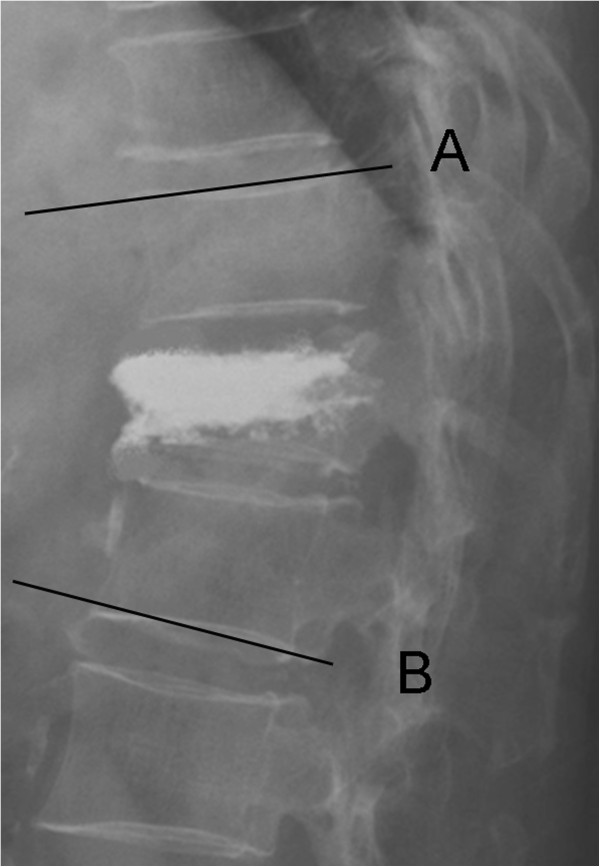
The balloon is deflated and withdrawn, and the resulting intravertebral cavity is filled with PMMA cement. Kyphotic angle defined as the Cobb angle measurements taken from the superior endplate of the vertebra one level above the treated vertebra (Line A) to the inferior endplate of the vertebral body one level below the treated vertebra (Line B) on the lateral X-ray image.
